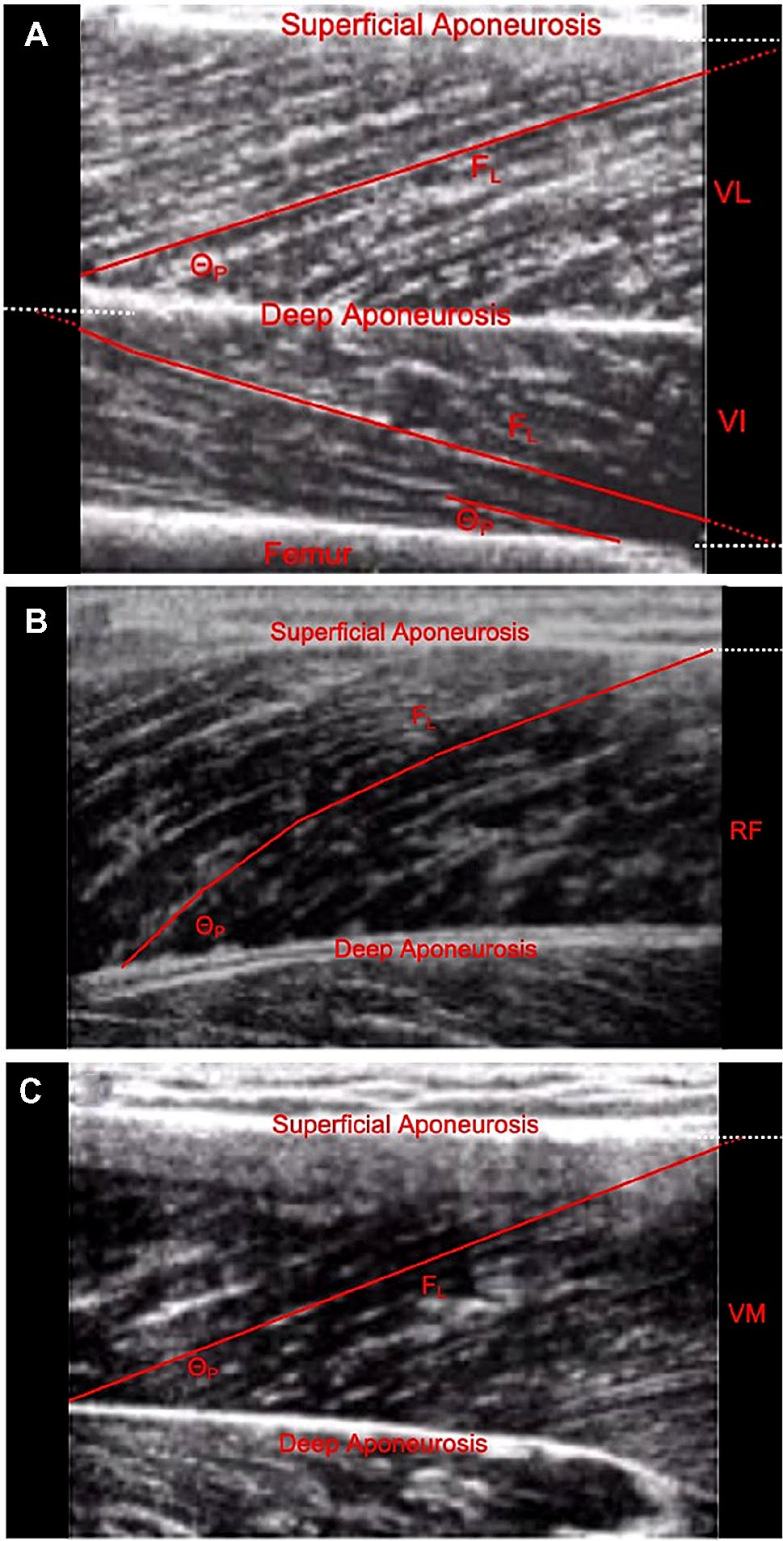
Representative ultrasound images and representation of the architectural assessment sites for a Vastus Lateralis (VL) and Intermedius (VI) (50% thigh length; VL_MID_, VI_MID_); b Rectus Femoris (RF_MID_) (55% thigh length) and c Vastus Medialis (VM) (40% thigh length: VM_PRX_). The dotted red lines show linear extrapolation for the measurement of fascicle length
